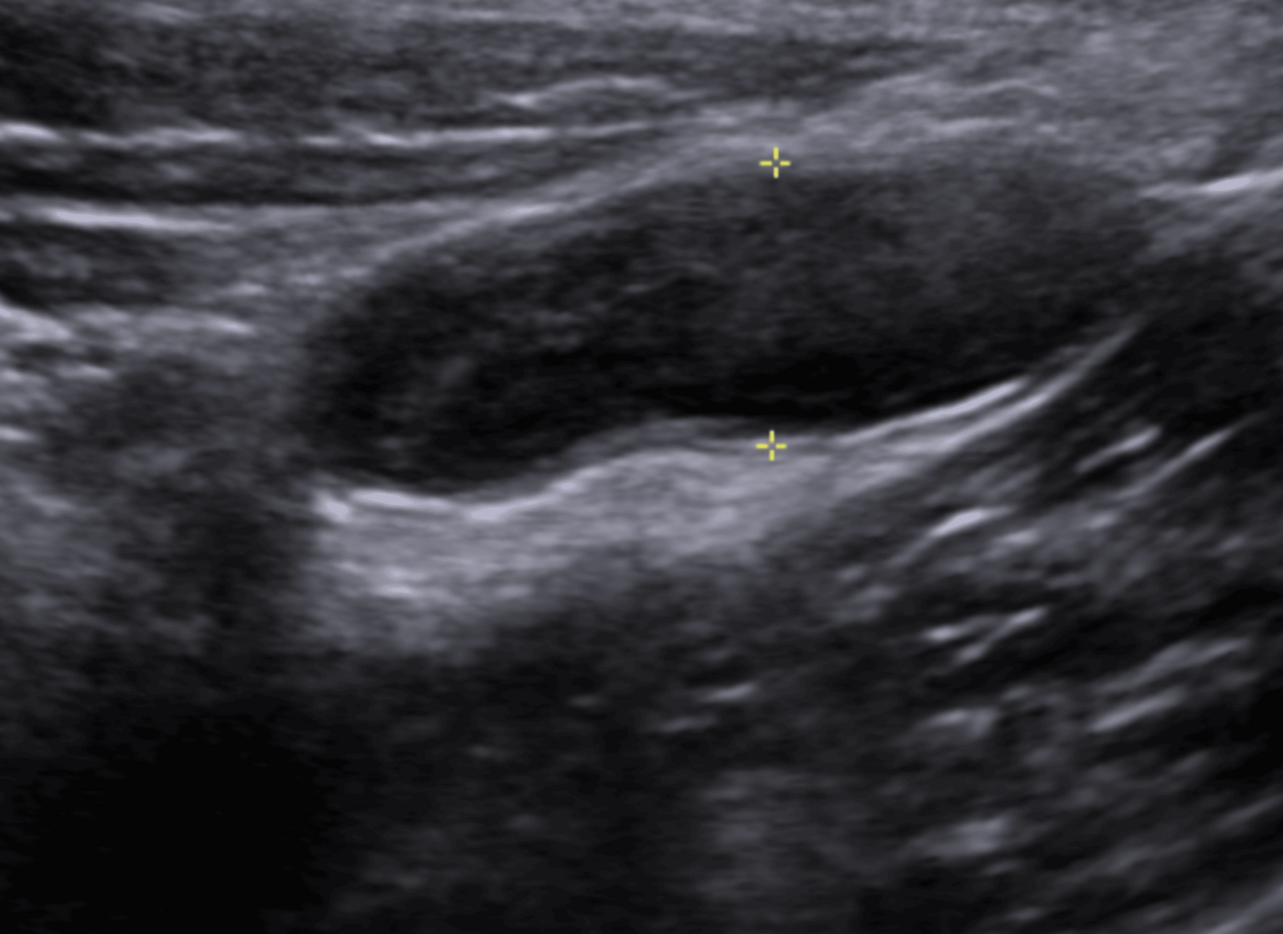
Abdominal ultrasound showing appendicitis Cecal appendix measuring 8.7 mm with free fluid and echogenic pericecal fat
